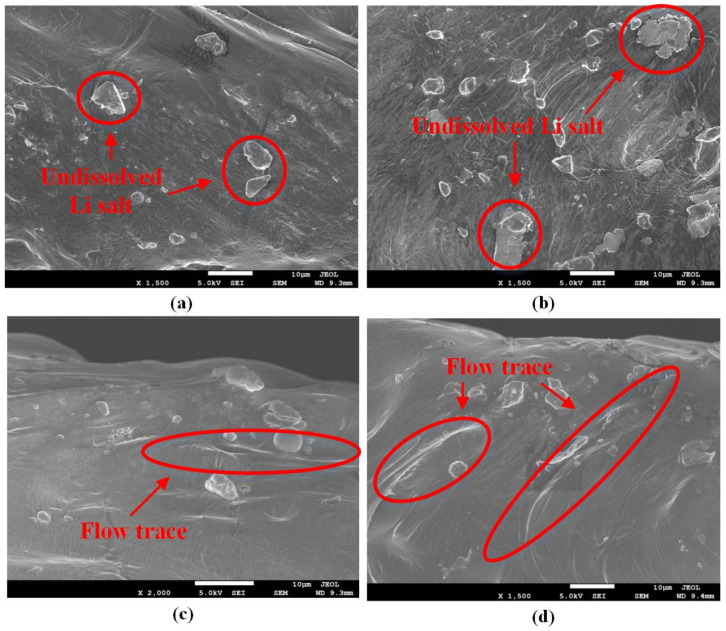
Surface morphologies of the electrolyte from: (a) prepared; (b) Reference Group; (c) Hot-pressed Group; (d) Ultrasonic Group.
